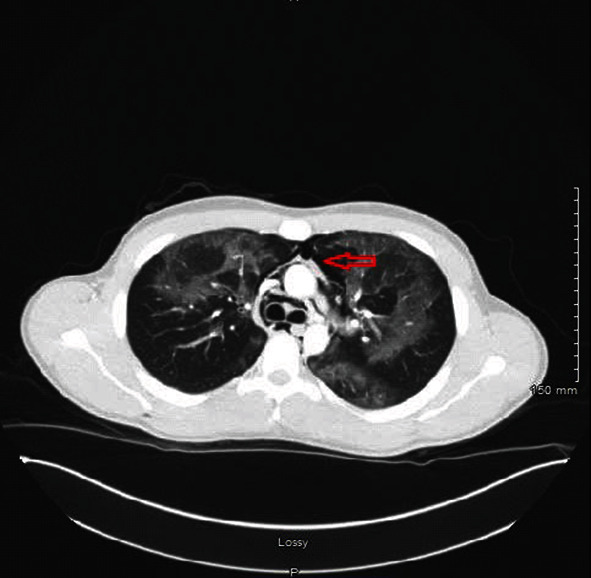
Computed tomography (CT) chest showing severe pneumomediastinum (red arrow) with diffuse pneumonitis.
